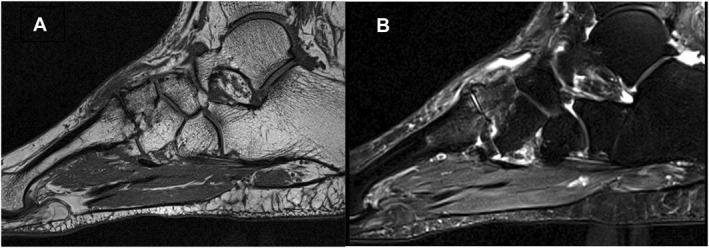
Sagittal plane T1 weighted (A) and STIR (B) MRI sequences show: Subchondral bone marrow oedema (grade 1) and dorsal osteophyte (grade 2) joint space narrowing (grade 2), bone erosion (grade1) at the intermediate cuneiform‐metatarsal joint. Small effusion at the tibio‐talar joint and talo‐navicular joint.
